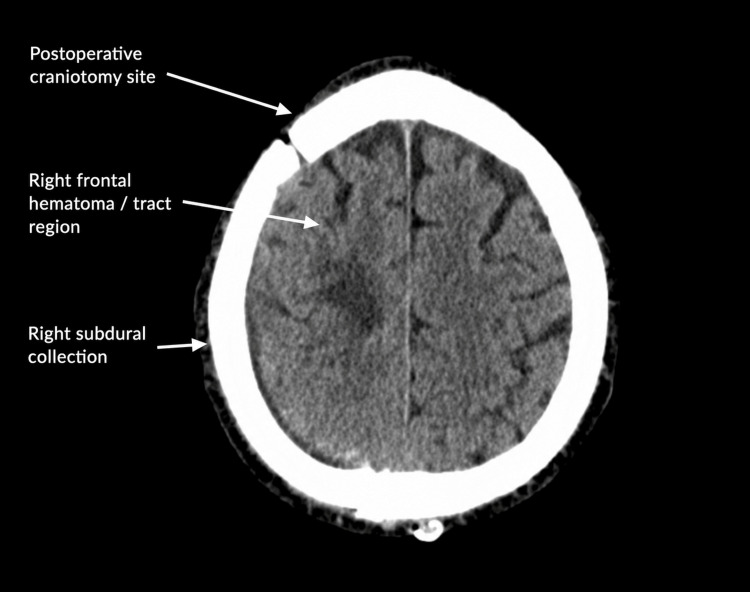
Postoperative day 25 CT demonstrating interval evolution of injury Noncontrast axial CT of the head on postoperative day 25 demonstrating postoperative craniotomy changes, expected interval evolution of the right frontal hematoma/tract region, and persistent but improving right convexity subdural collection. No new acute intracranial abnormality was identified
